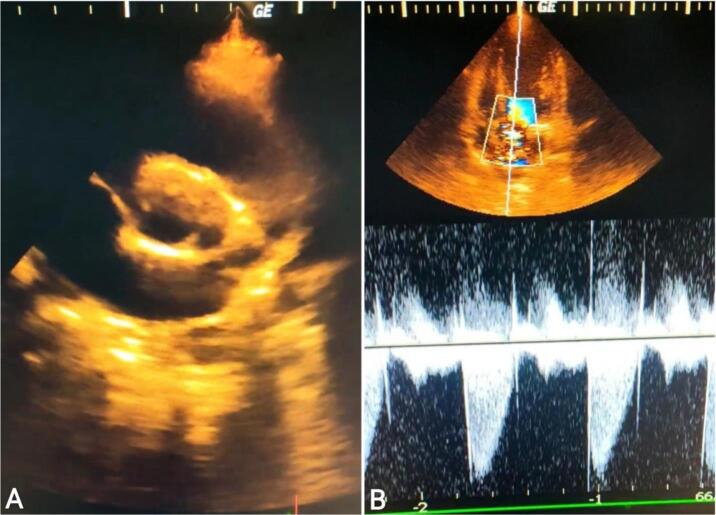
Image (A): Transthoracic short-axis view displays a bicuspid aortic valve. Image (B): Continuous wave doppler across the aortic valve shows oderate aortic stenosis with Vmax at 3.5 m/s.
